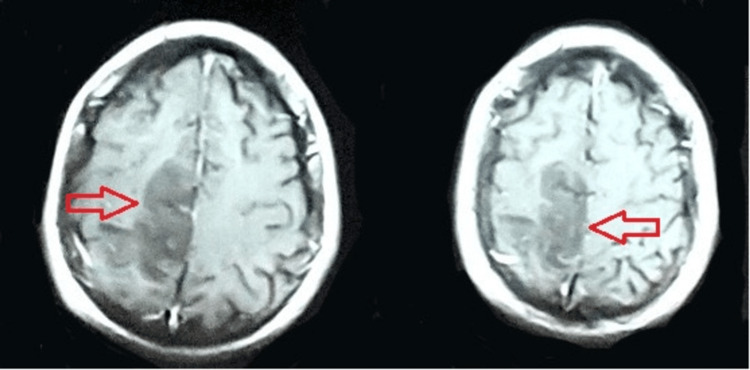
T1W MRI brain T1W MRI shows a hypo-intense lesion in the frontoparietal region. T1WI: T1 weighted image
